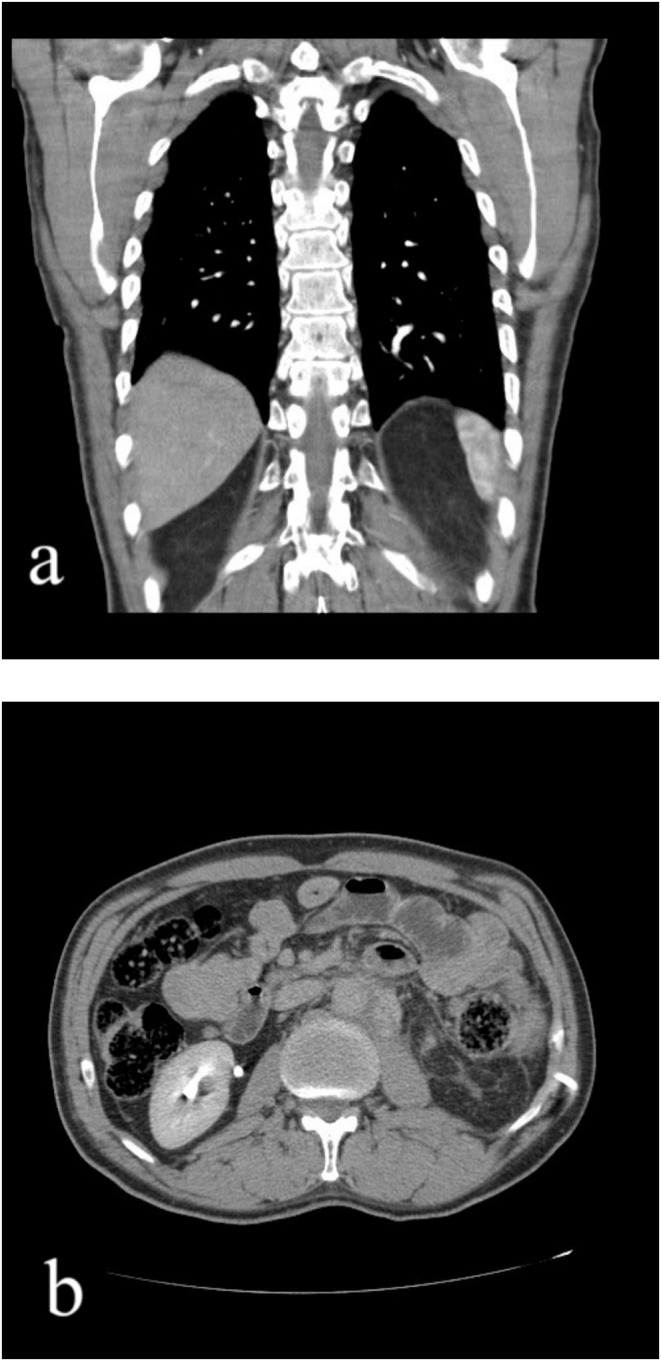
A chest‐abdominal and pelvis CT in coronal view (a) and axial view (b) showing a residual mass of about 11 × 7 × 4 cm with a partial response to radiotherapy, without distant metastasis.
